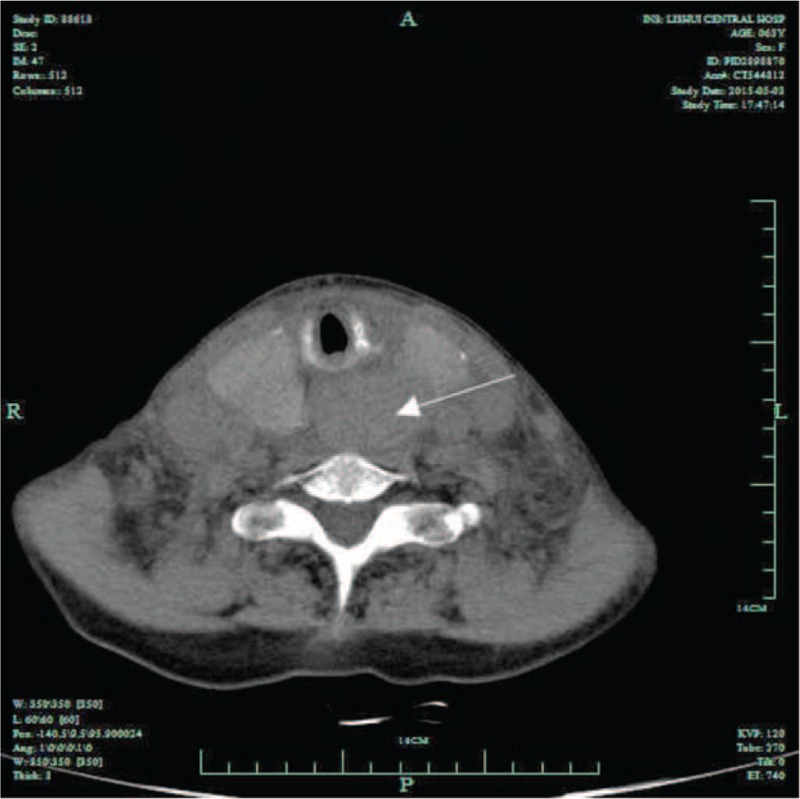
CT showed a well-defined mass compressing the trachea and esophagus.
